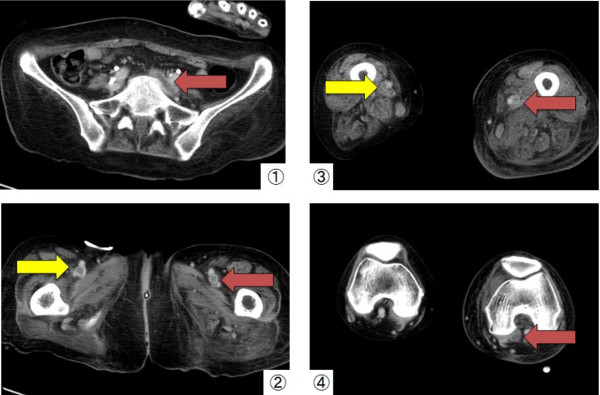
Contrast-enhanced CT scans of the lower limbs. Contrast-enhanced CT scans revealed a lower extremity deep vein thrombosis involving the left common iliac vein (1), bilateral femoral veins (2, 3), and the left popliteal vein (4).
